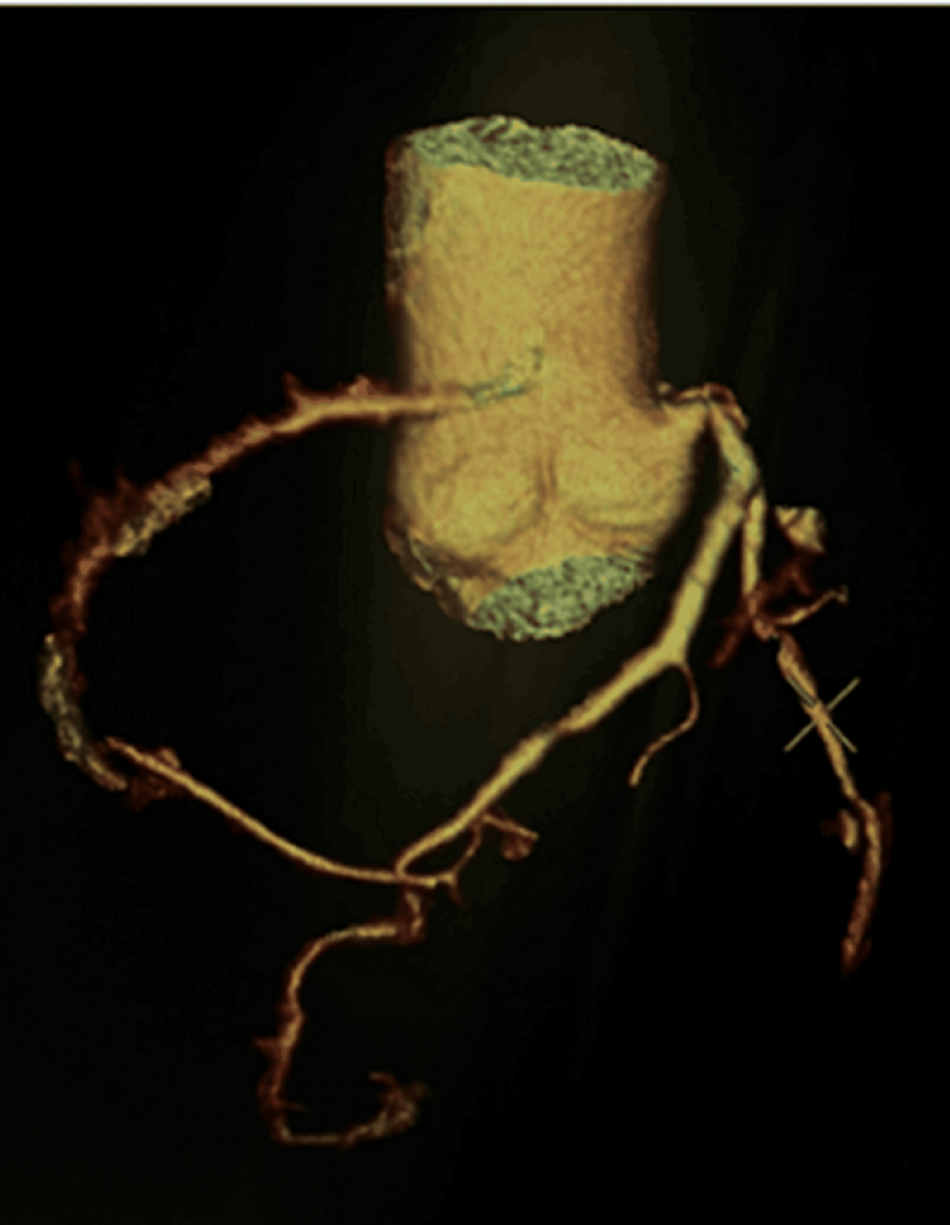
CT coronary angiogram: preoperative three-dimensional reconstruction of an anomalous RCA origin from the left coronary sinus of Valsalva in an isolated aortic view CT, computed tomography; RCA, right coronary artery
